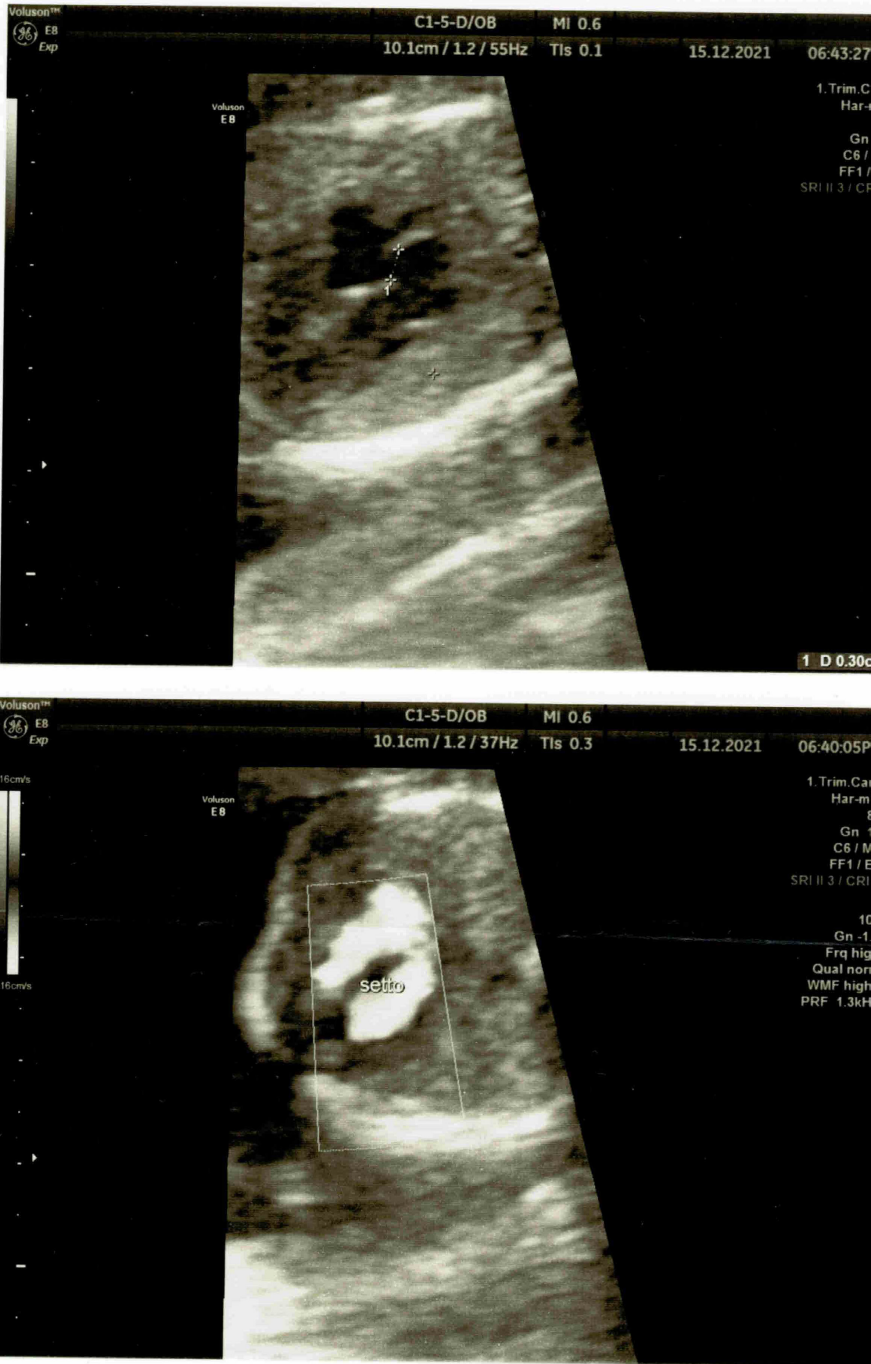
The fetus was small for gestational age, and cardiac anomaly was evidenced consisting in large foramen ovale with suspected ostium primum defect, small penis, altered uterine Doppler velocimetry (RI 0.92) with protodiastolic notch and absent diastolic flow at umbilical artery velocimetry.
